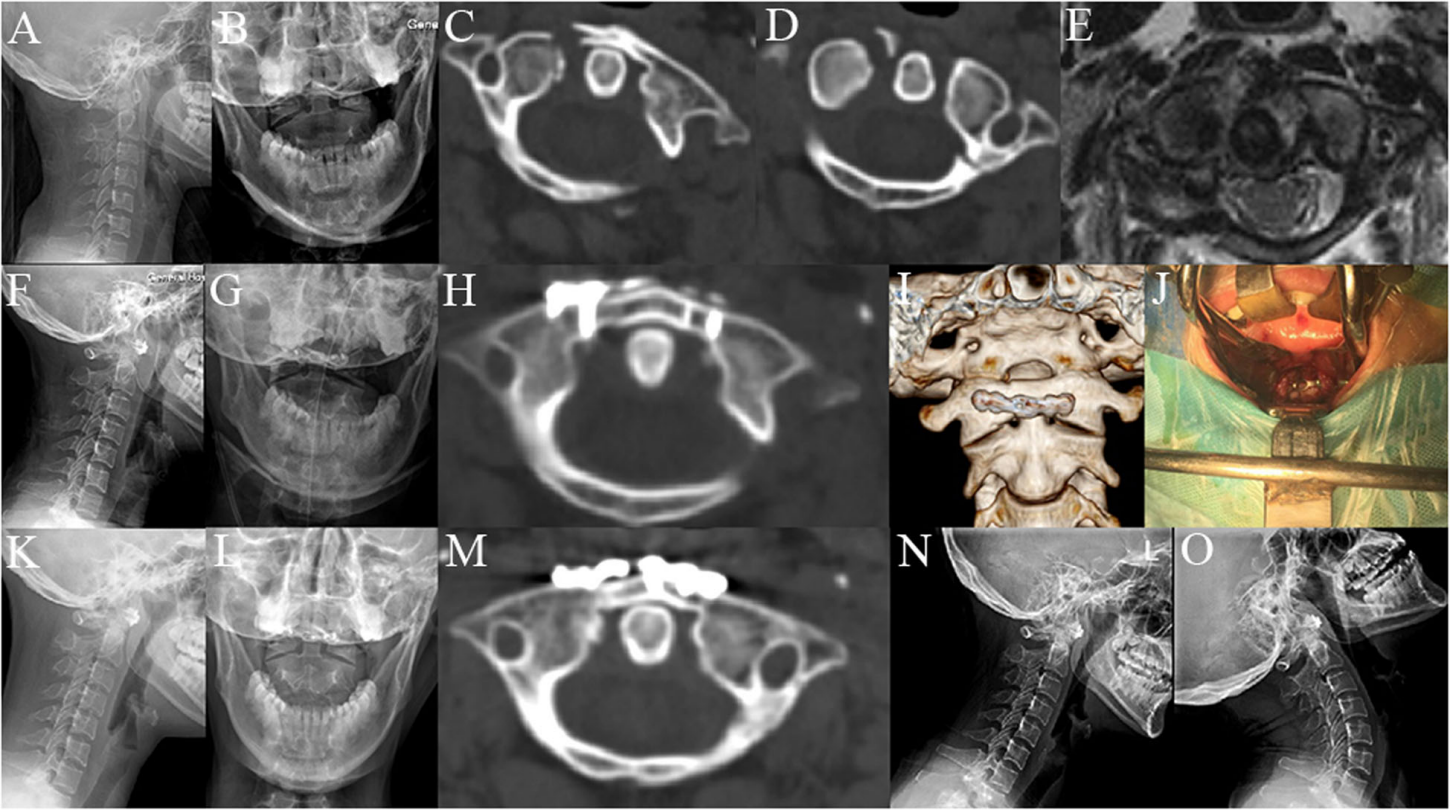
A 32-year-old female with atlas fracture treated by transoral anterior C1-ring osteosynthesis using a laminoplasty plate. Preoperative cervical lateral (a) and open-mouth X-rays (b), axial CT (c, d) and MRI images (e) showed combined fracture of the anterior and posterior arches with type I TAL injury. Postoperative cervical lateral (f) and open-mouth X-rays (g) identified the relatively good C1–C2 alignment. Axial CT (h) and the reconstructed images (i) after surgery showed reduction of the anterior arch fracture and optimal plate location. Transoral intraoperative view (j) showed good position of anterior laminoplasty plate fixation. Cervical lateral (k) and open-mouth X-rays (l) at 6 months after surgery showed stable fixation. An axial CT image (m) at 6 months after surgery indicated solid bone union. The dynamic cervical X-rays (n, o) at final follow-up revealed no sign of instability of C1–C2 and no loosening of the fixation
